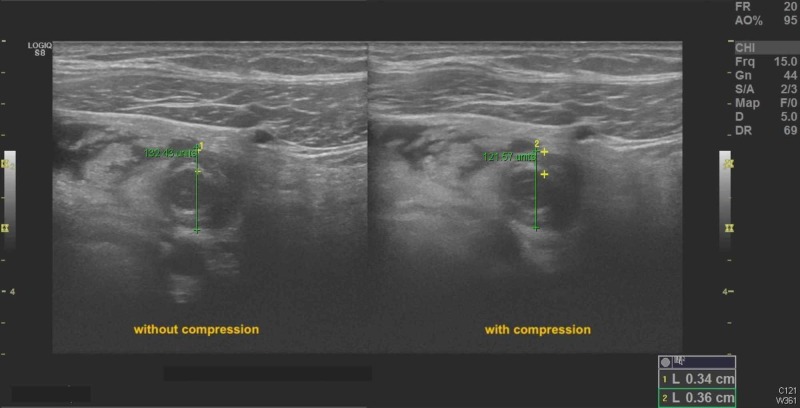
Sonogram demonstrating minimal compressibility of appendix on transverse view. Note the depth of the noncompressed and compressed appendix are almost equal.
